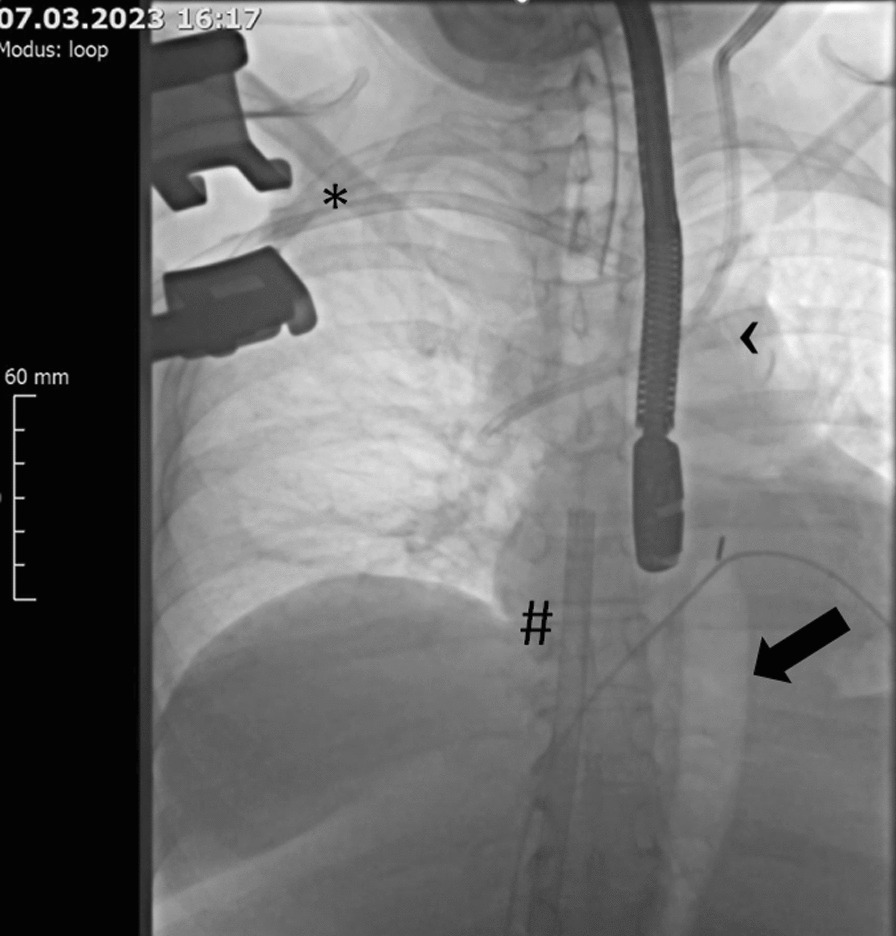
Chest X-ray showing veno-arterial extracorporeal life support and intra-aortic balloon pump simultaneously implanted via the right axillary artery. Arrow—inflated intra-aortic balloon pump. Arrowhead—intra-aortic balloon pump catheter leading to the right axillary artery. *Arterial cannula of the veno-arterial extracorporeal life support device implanted via the right axillary artery. # Venous cannula of the veno-arterial extracorporeal life support device implanted via the femoral vein
